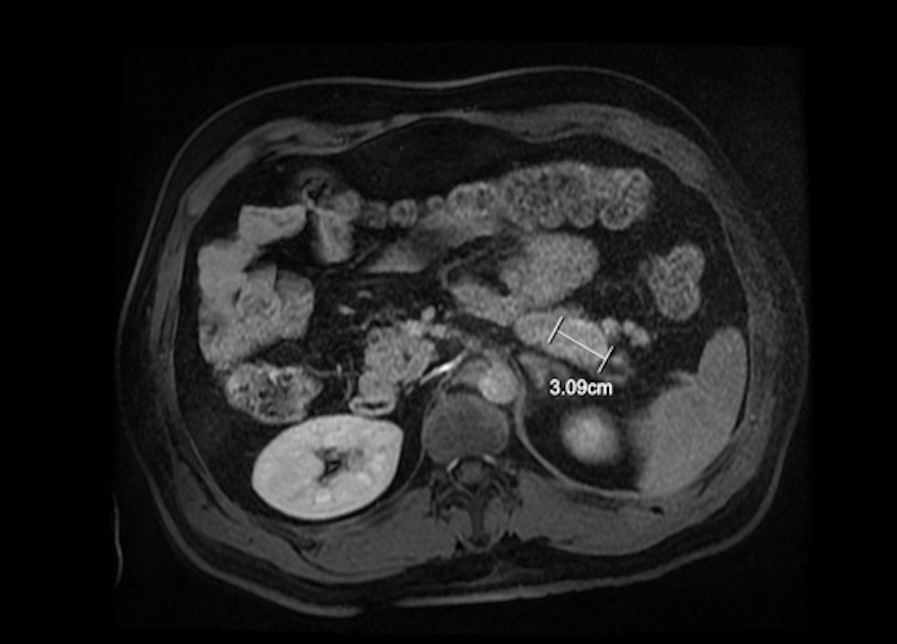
T1 axial post-gadolinium dynamic three-dimensional magnetic resonance imaging of abdomen showing an intrapancreatic lesion of 3 × 2 cm.
